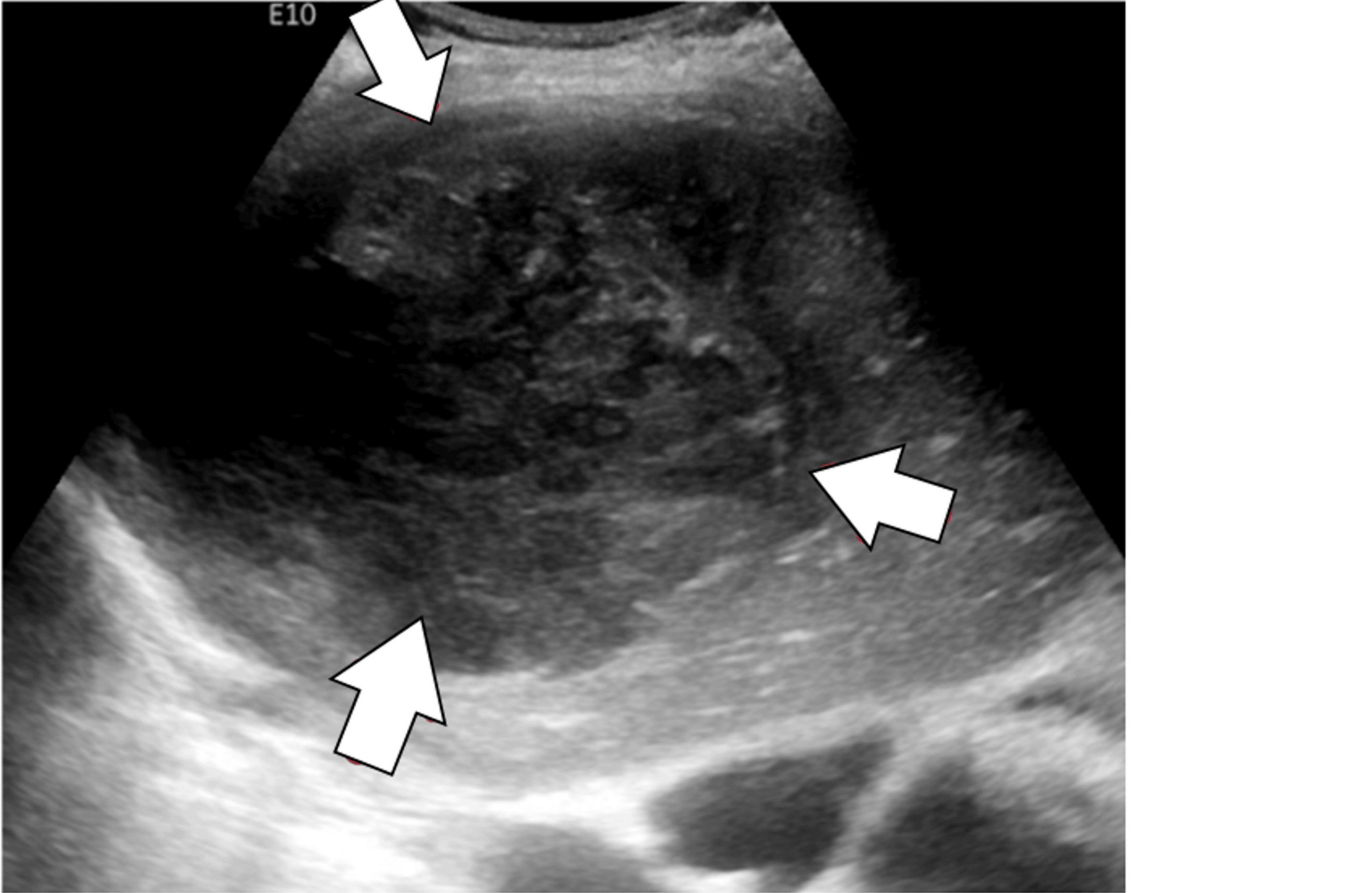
Ultrasound of the abdomen identified a solid mass within the right hepatic lobe near the dome of the liver measuring 12.9 x 9.8 x 9.6 cm, concerning for underlying neoplasm
